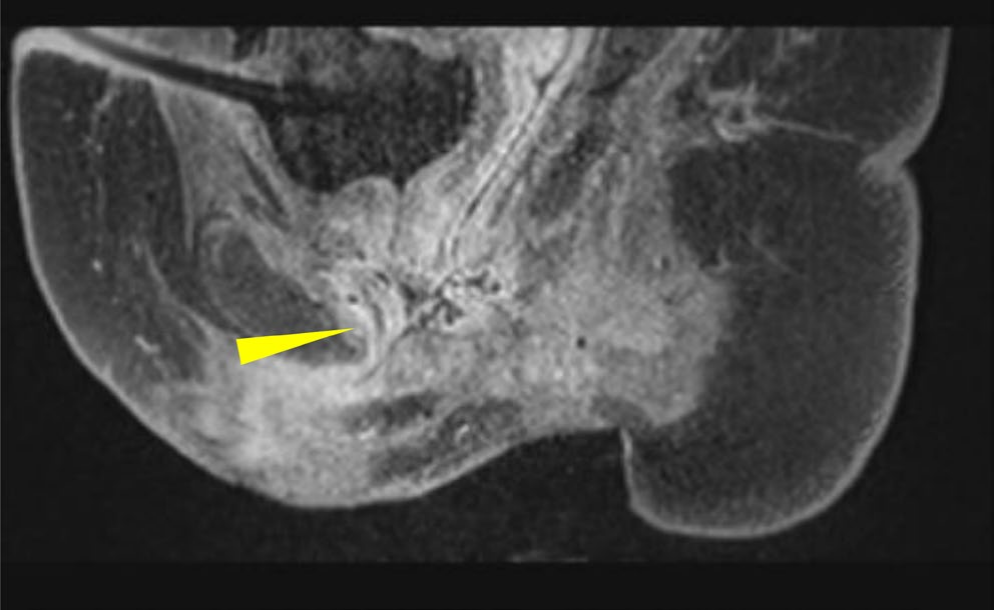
MRI image showing complete mid‐urethra transection and displacement (yellow arrow: Mid‐urethra transection).
